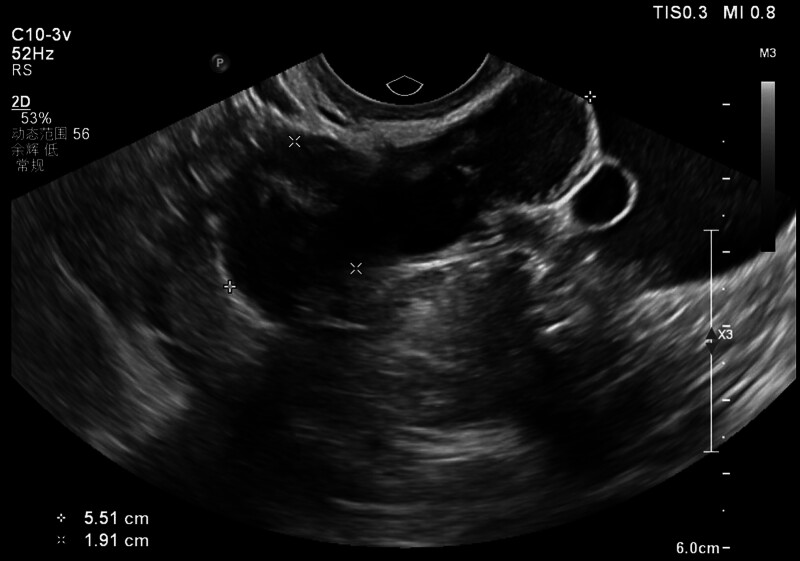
Transvaginal ultrasonography demonstrated a heterogeneous hyperechoic adnexal mass (5.51 × 1.91 cm) in the right pelvic region.
